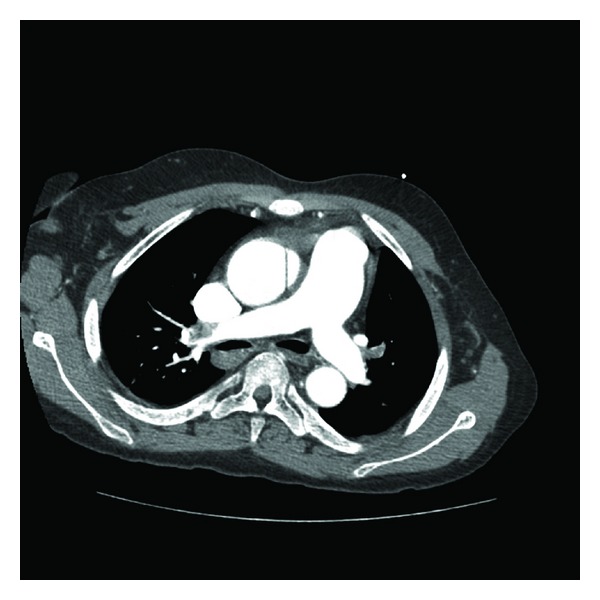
CT scan showing large filling defect in main pulmonary artery.
